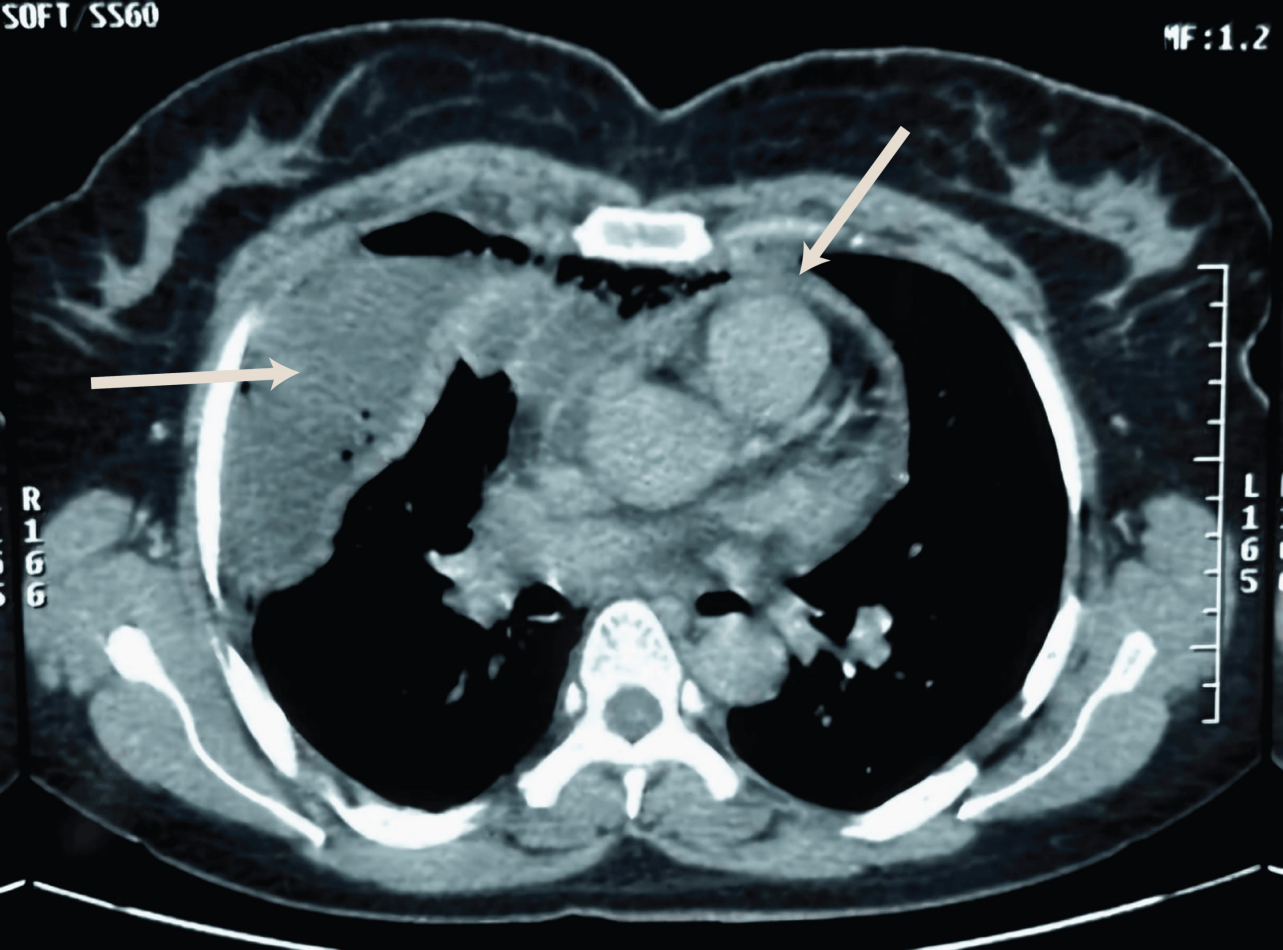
Axial thoracic CT, mediastinal window, showing right pleural empyema and a thin pericardial effusion The right arrow indicates the pleural empyema, and the left arrow indicates the pericardial effusion.
